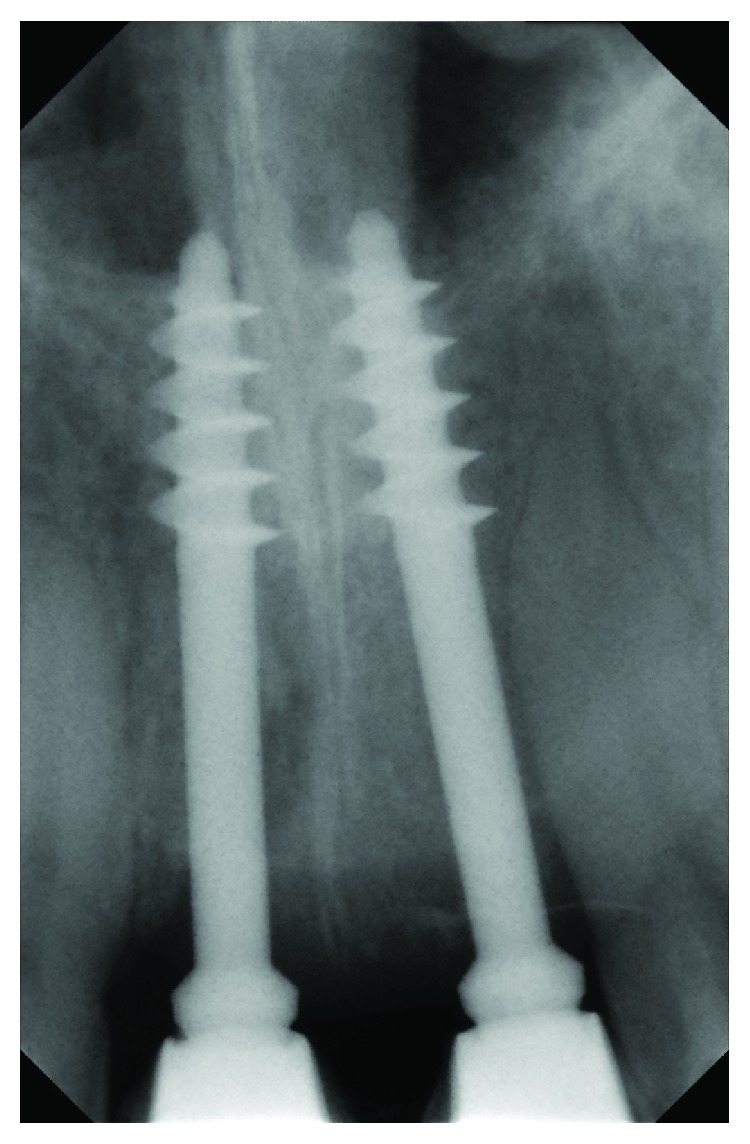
X-ray after 3 months with try-in metal-ceramic crowns.
